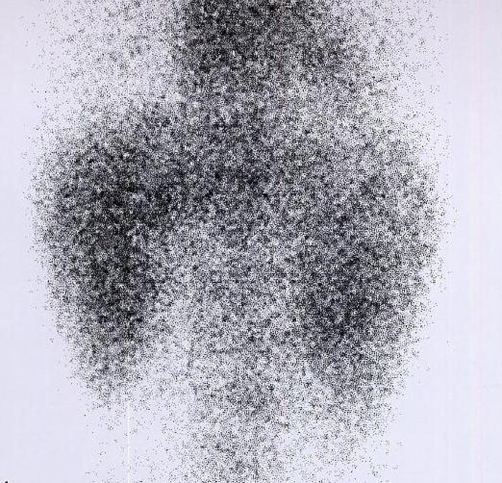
^99m^Tc-DMSA renal scintigraphy of the patient while on enalapril. The posterior image shows decreased radiotracer uptake in both kidneys. Radiotracer uptake in the liver and background is increased. This pattern is suggestive of renal failure.
